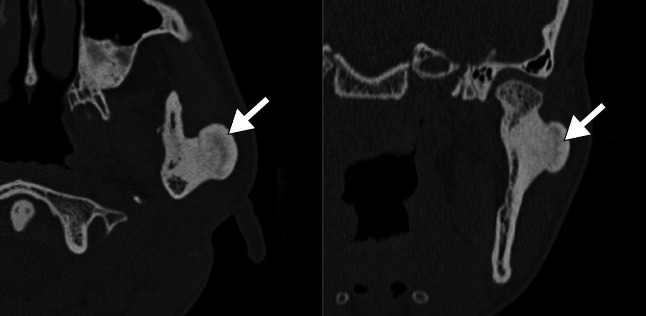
Osteoma in a 19-year-old female patient with Gardner’s syndrome. CT
